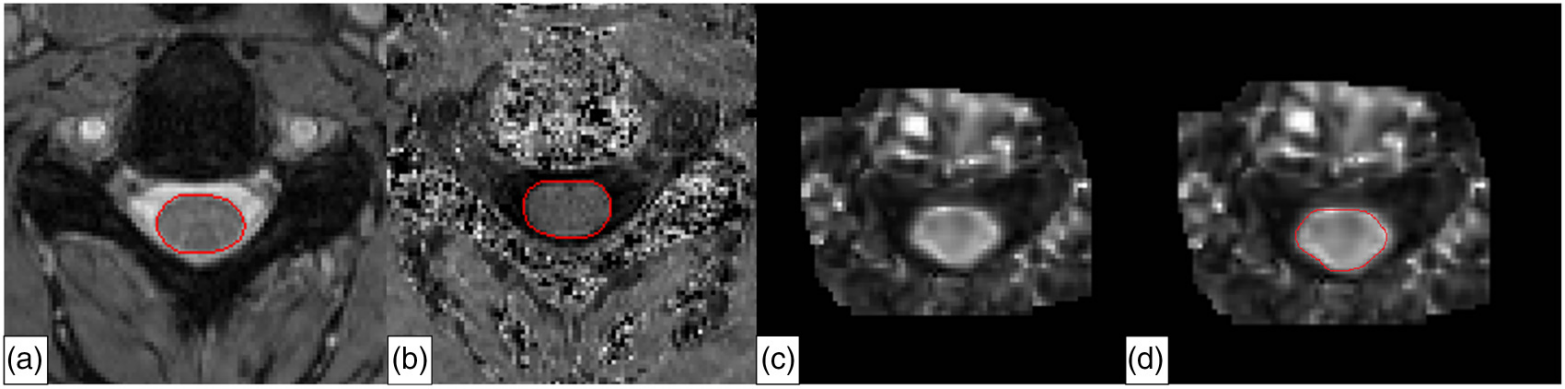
Quantitative spinal cord MRI analyses (a) Manual segmentation of spinal cord cross-sectional area on the MTon map; (b) Manual segmentation of region-of-interest (ROI) on magnetization-transfer ratio (MTR) map; (c) fractional anisotropy (FA) map; DManual segmentation of ROI on FA map.
